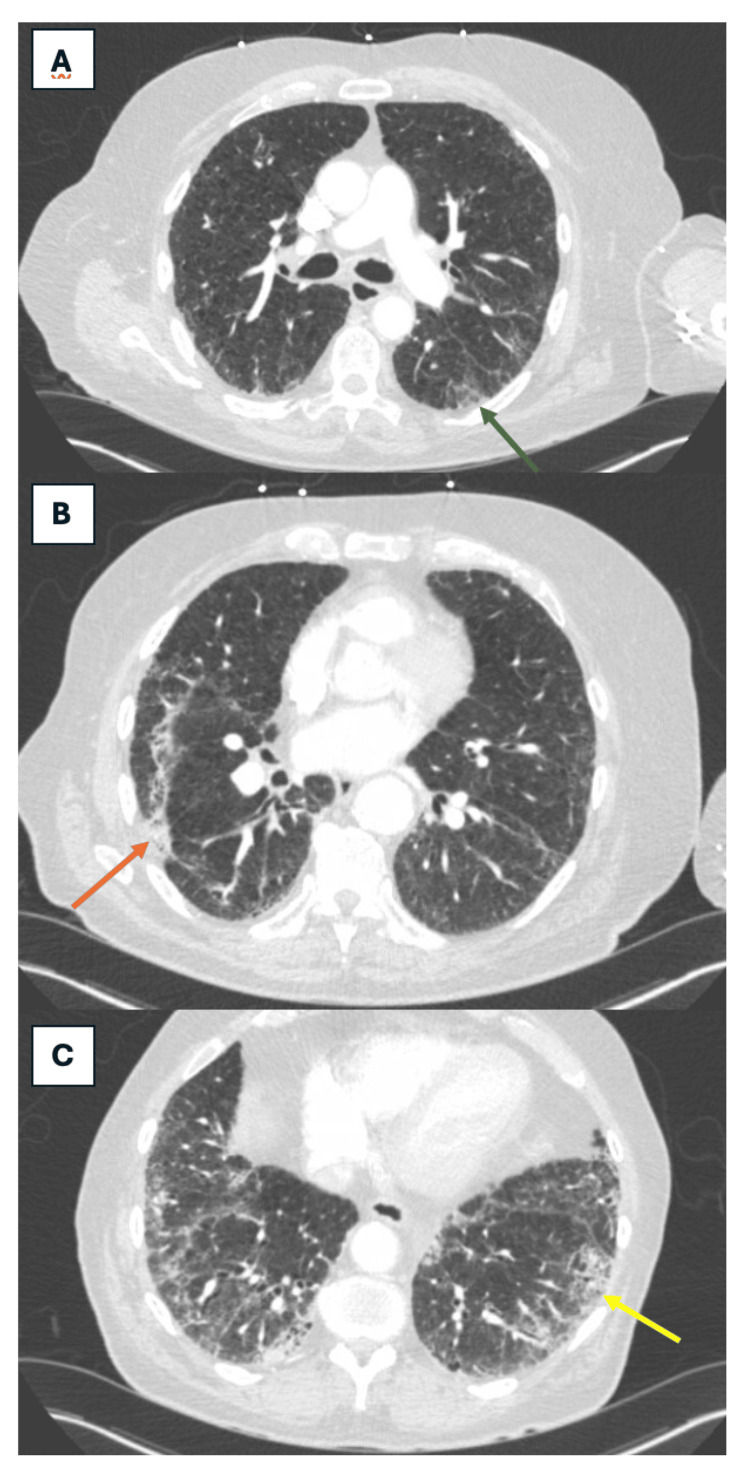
One-year follow-up CT imaging: Case 2 (A) Dark green arrow depicting new ground-glass opacities. (B) Orange arrow pointing to the progression of reticular opacities and fibrosis, along with ground-glass opacities. (C) Yellow arrow showcasing subpleural reticulations as well as septal thickening.
